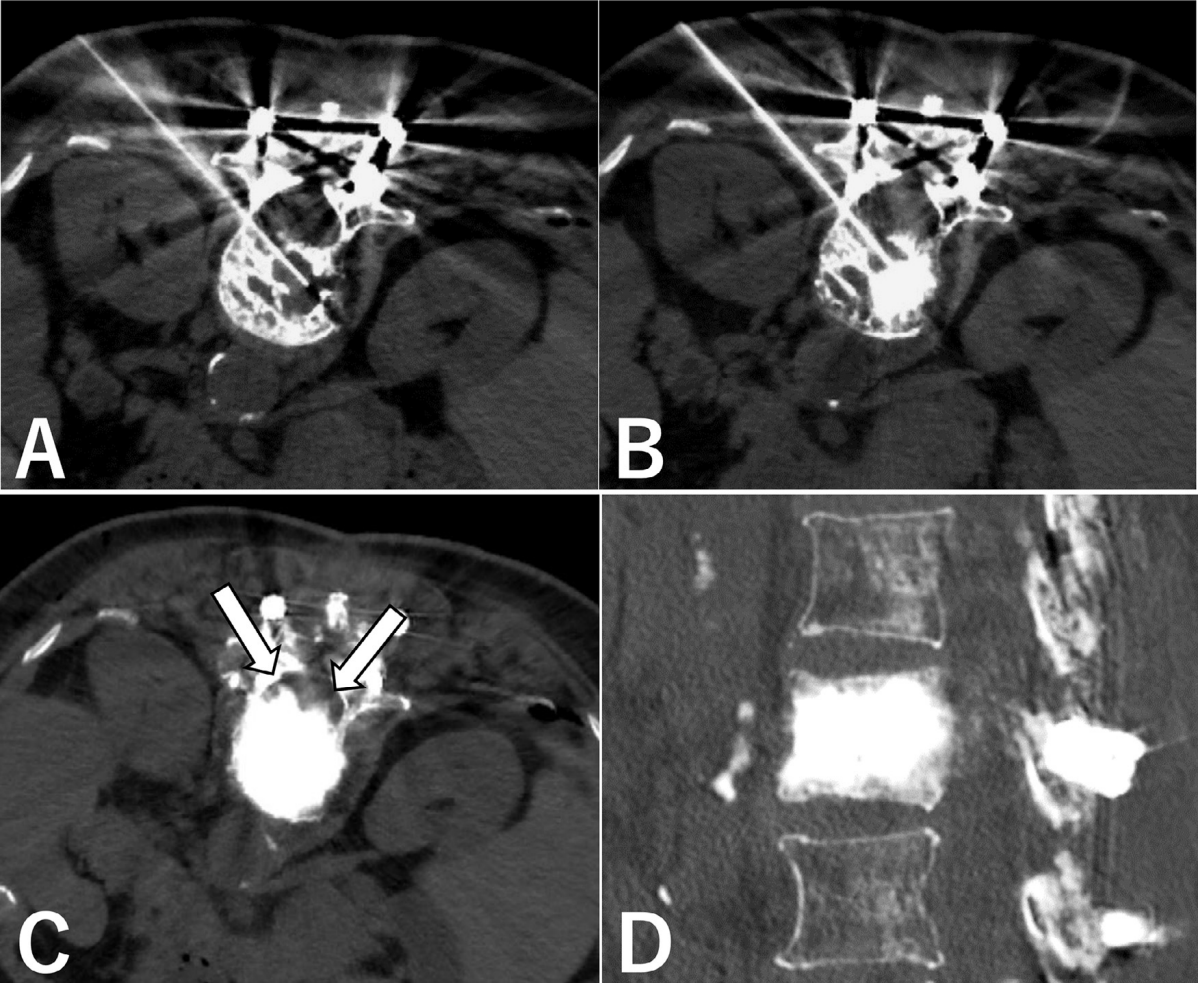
Fluoroscopy and CT-guided intraosseous sclerotherapy. (A) A puncture needle was introduced within the vertebral body by a posterolateral approach. (B) Five percent ethanolamine oleate mixed with contrast media was injected into the lesion. (C, D) Sclerosant is well distributed to the epidural component (arrows) and in the entire vertebral lesion on postoperative CT image. CT, computed tomography.
